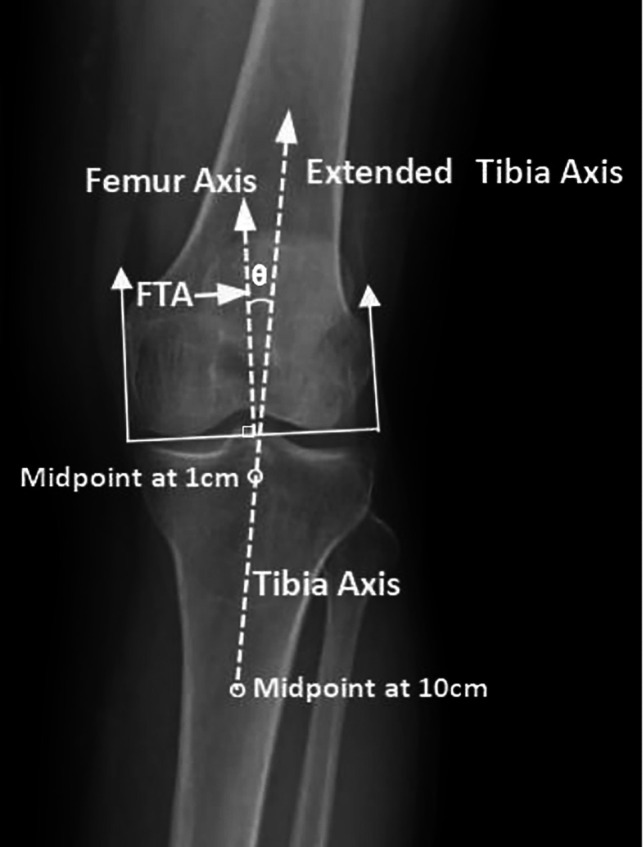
Measurement of femorotibial alignment with a short knee radiograph.
